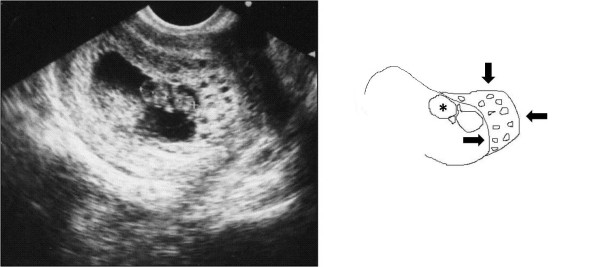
Transvaginal ultrasound study. An apparently normal fetus (asterisk) and villi with multiple hypoechoic cysts (arrows) at 8 weeks' gestation. Crown-rump length is 20 millimeters. Each cyst is 2-3 millimeters in diameter.
